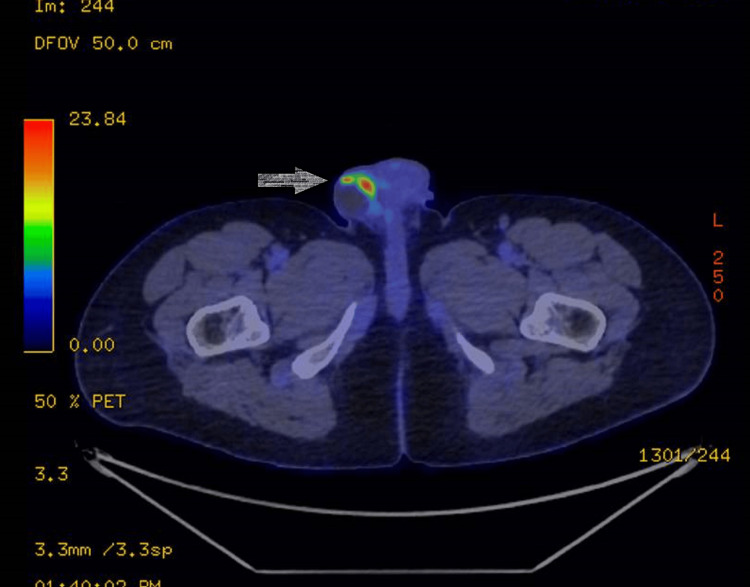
Whole-body FDG PET CT scan showing complex, partly-cystic, partly-solid mass at the right scrotum with intense FDG accumulation within solid components FDG: Fluorodeoxyglucose, PET: positron emission tomography, CT: computed tomography
